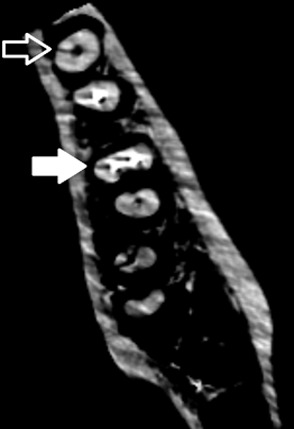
An axial section of CBCT shows a fracture in a non-filled root (the hollow arrow) and a streaking artifact mimics the fracture line in a root canal filled with gutta-percha (the solid arrow)
